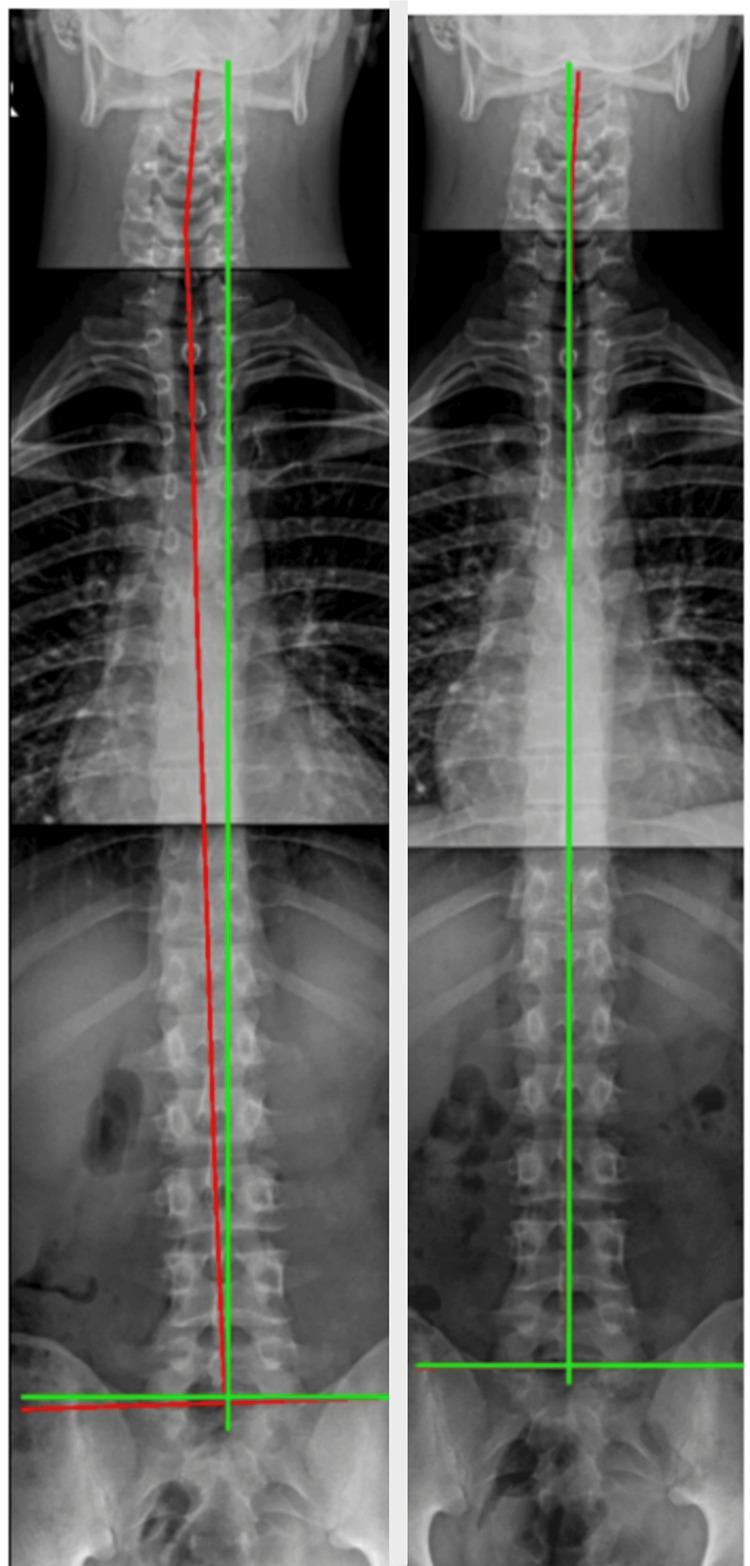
Full-spine sectional stitched anterior-posterior radiograph The initial evaluation and follow-up anterior-posterior radiograph demonstrated post-treatment improvement in the coronal balance and spine alignment. The red line represents a centroid measurement of the vertebral bodies before treatment on the left and post-treatment on the right. The green line represents the ideal skeletal coronal and spine alignment
